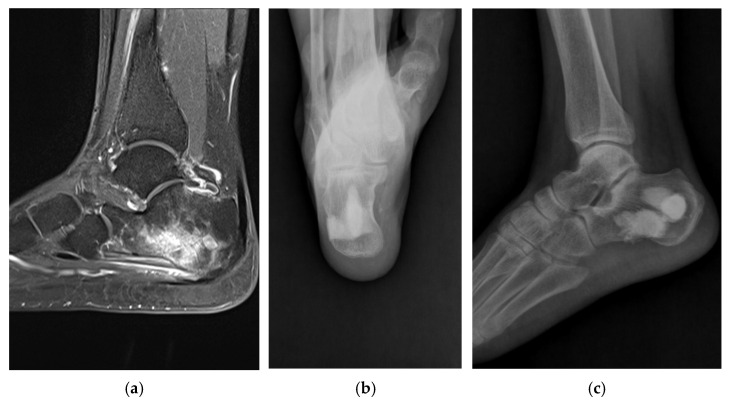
Antibiotic-mixed cement filling in a 57-year-old female patient (identified pathogen: Salmonella typhi). (a) Sagittal MRI shows chronic osteomyelitis on the calcaneus preoperatively. (b) Calcaneus axial radiograph shows that antibiotic-impregnated cement filling is well maintained 3 years postoperatively. (c) Calcaneus lateral radiograph shows that antibiotic-impregnated cement filling is well maintained 3 years postoperatively.
